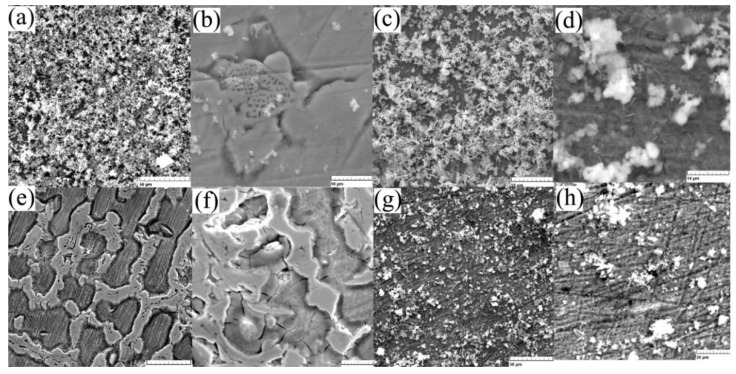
SEM images of the surface: (a) C sample after 1 day immersion (1 kx) and (b) after ultrasound cleaning (5 kx), (c) W sample after 1 day immersion (1 kx) and (d) after ultrasound cleaning (5 kx), (e) C sample after 7 days immersion (1 kx), and (f) after ultrasound cleaning (2 kx), (g) W sample after 7 days immersion (1 kx) and (h) after ultrasound cleaning (2 kx).
